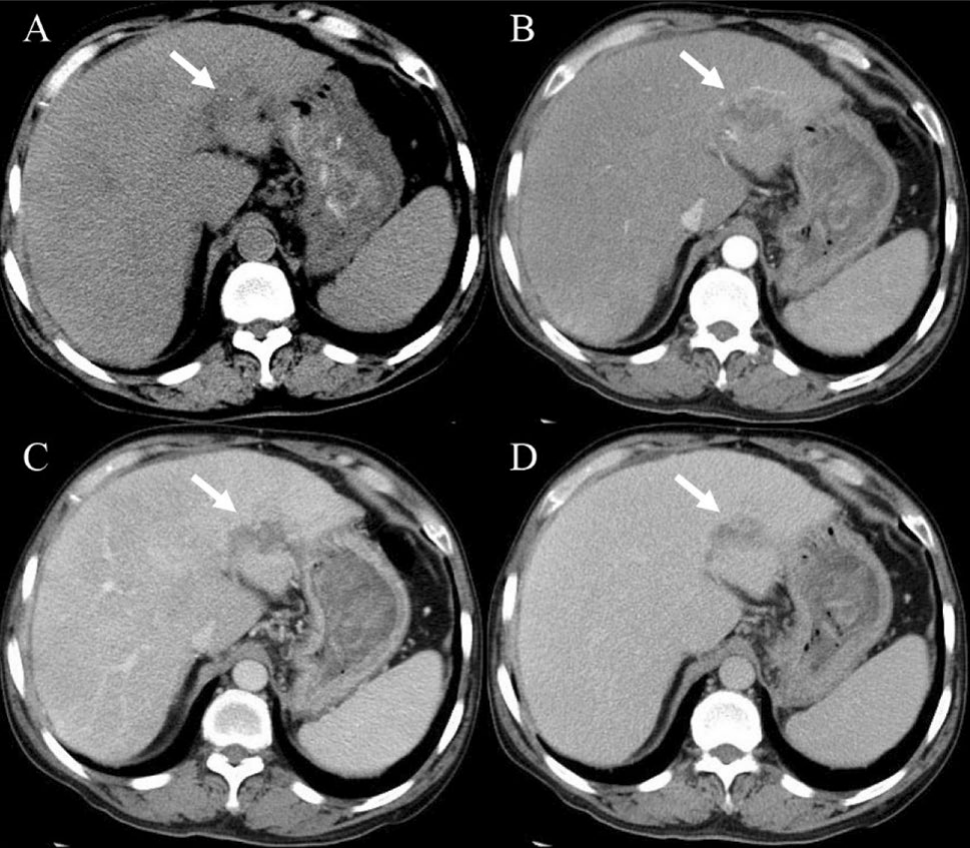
A case of hepatic sclerosed hemangioma mimicking intrahepatic cholangiocarcinoma (man, 63-years-old). Plain CT revealed a slightly low-density mass with dot-like calcification and irregular margin in segment 2 of the liver (A). The mass showed peripheral heterogeneous enhancement in the arterial phase (B), internal non-enhancement in the portal venous phase (C) and delayed phase (D) (arrows)
